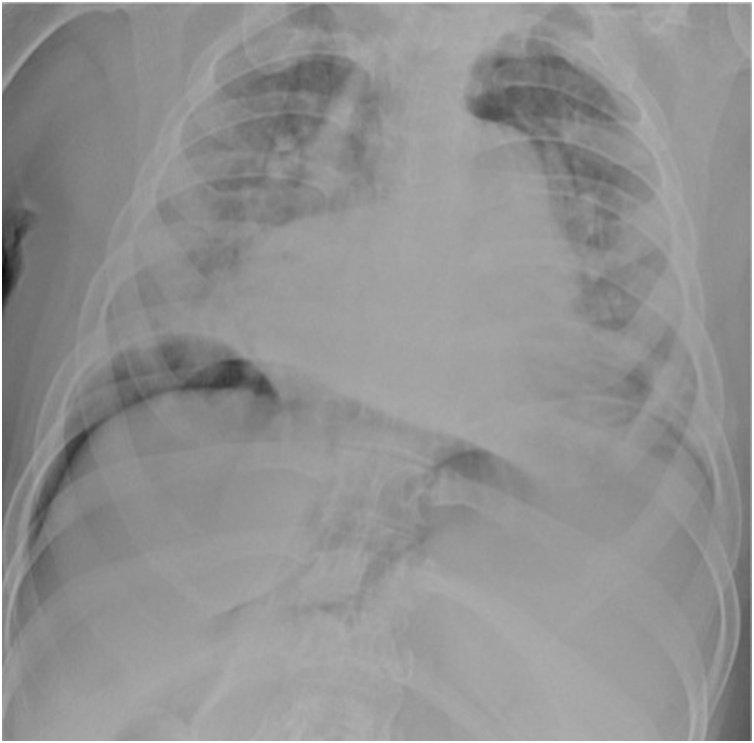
Pneumoperitoneum evidenced on abdominal radiograph.
